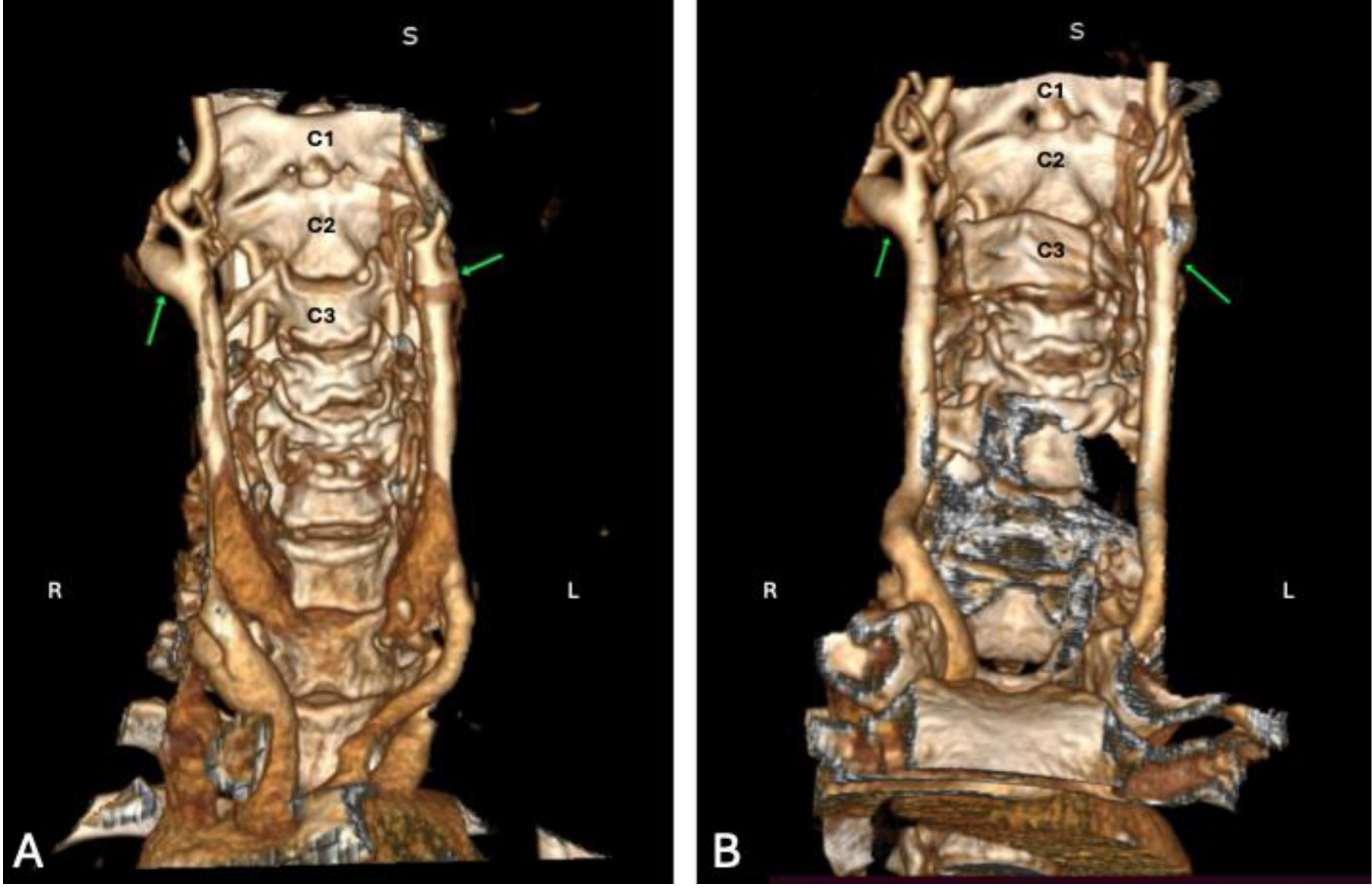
3D Rendered Image of Computed Tomography Head and Neck Angiography (CT Angiography). This 3D-rendered image depicts two distinct cases (A and B) of bilateral high carotid bifurcation (the green arrow). In both instances, the right-sided bifurcation occurs at the level of the C2 vertebra, while the left-sided bifurcation is situated between the C2 and C3 vertebrae. Note. S – Superior; R – Right side; L – Left side.
